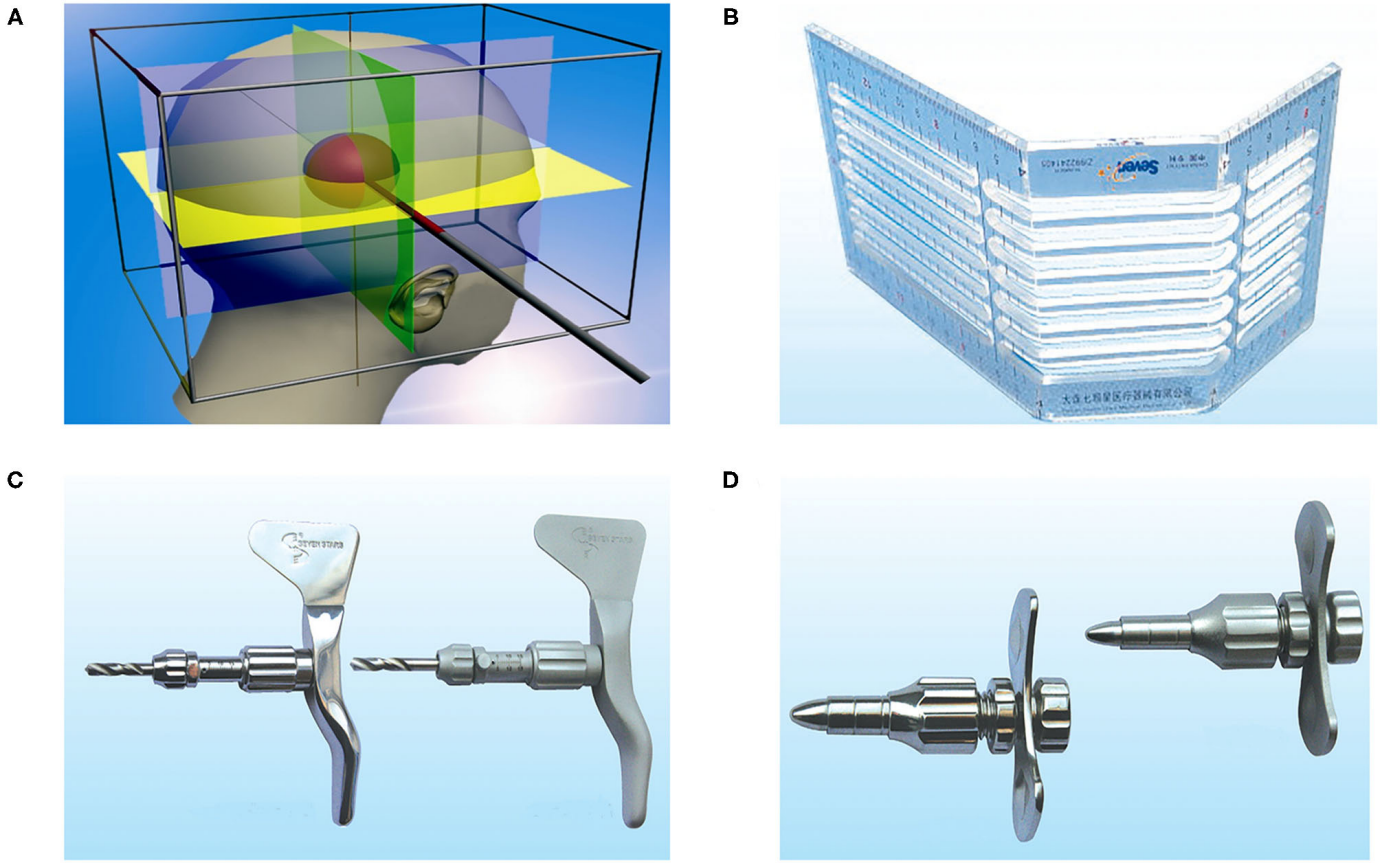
The principle and devices of cubic oriented stereotactic aspiration technology. (A) The principle of cubic oriented stereotactic aspiration: according to the principle of solid geometry, the position of any point in space can be determined by the three-dimensional directional coordinate system. Combining this principle with cranial anatomy, the “quasi-circular” head is “framed” in cube space. According to the principle that three mutually perpendicular planes intersect to form a three line and a point in a cube, a horizontal plane, a coronal plane, and a sagittal plane passing through the point can be made, respectively, to form a three-dimensional directional coordinate system with this point as the origin. According to the parameters of CT scanning, the vertical projection lines and planes in the forehead, temporal part, and top and occipital parts were determined. Then, the center of the hematoma was determined according to the intersection of the three planes. The straight line formed by the intersection of any two planes in the three mutually perpendicular planes can be used as the puncture path and the position of the other plane can determine the puncture depth. This allows accurate access to the center of the hematoma. The stereotactic ruler (B), skull drill (C), and skull keyhole tool (D) are used in cubic oriented stereotactic aspiration.
